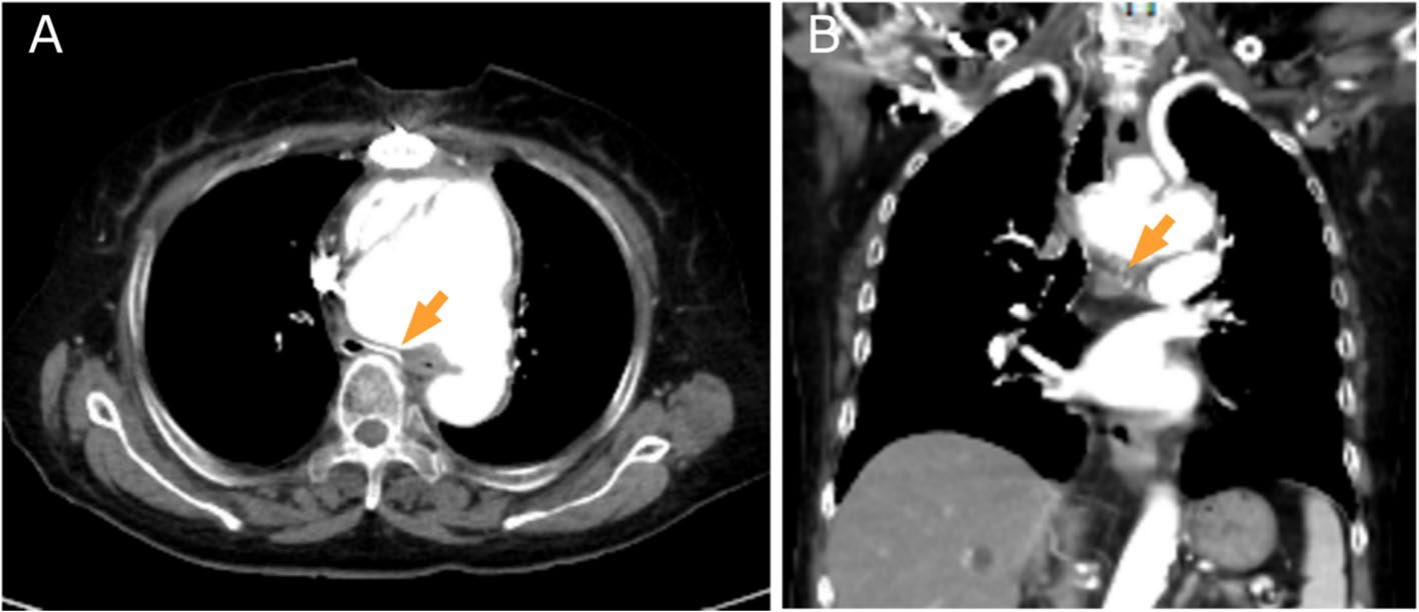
CT image of the chest (A transverse plane, B coronal plane) on day Z + 1. The images show dilation of the aortic arch (maximum diameter: 86 mm). The trachea is compressed and narrowed (arrows) by the aneurysm and the vertebral body above the bifurcation
